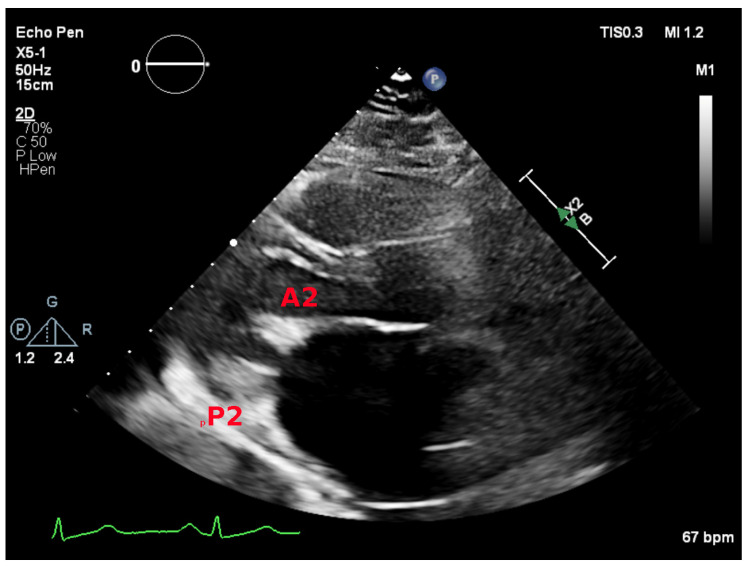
Parasternal long-axis view showing the A2 and P2 mitral scallops.
